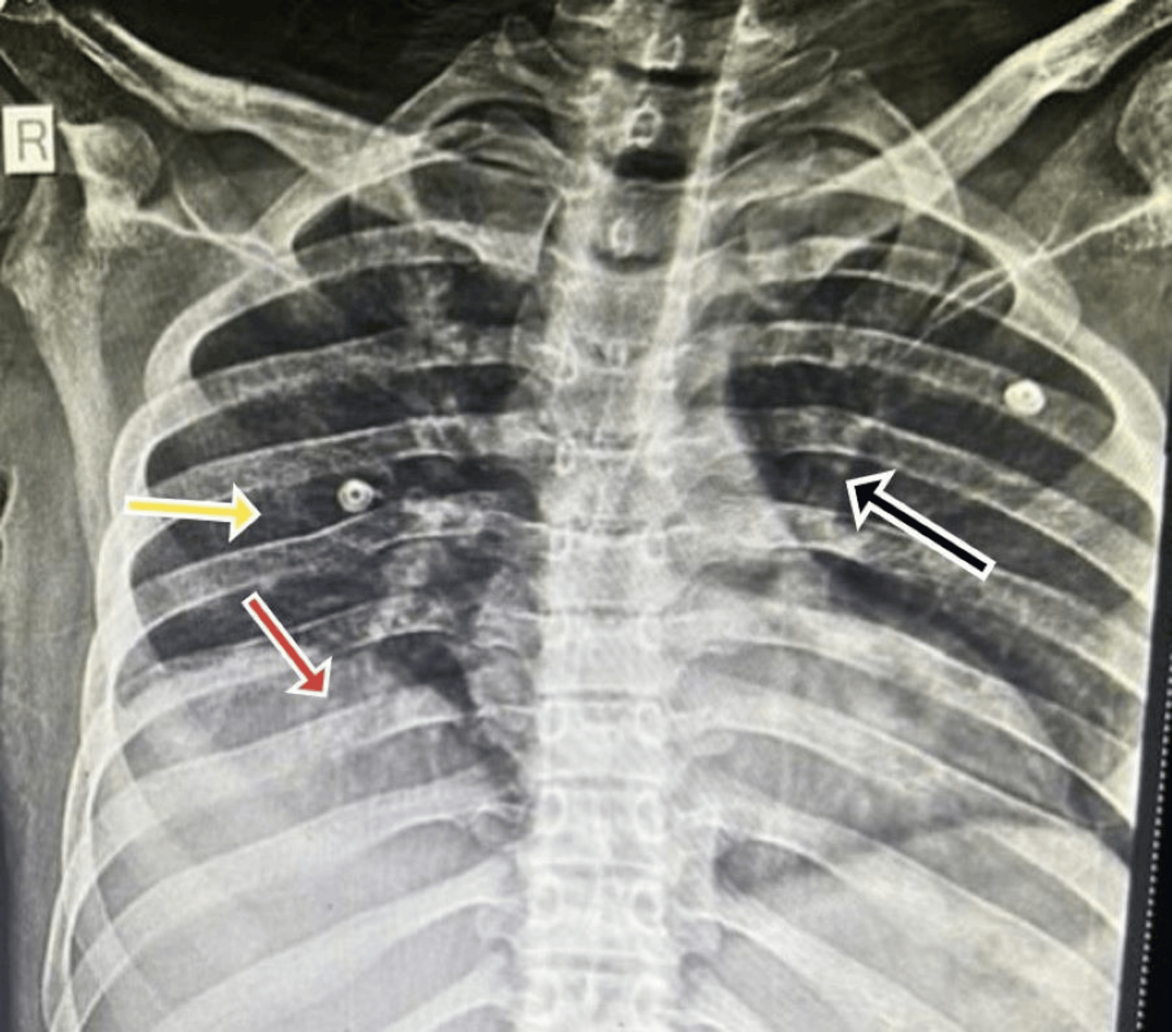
Chest X-ray showing bilateral interstitial infiltrates (black arrow), blunting of the Costo-phrenic angle indicating pleural effusion (red arrow), and pulmonary fibrosis (yellow arrow).
